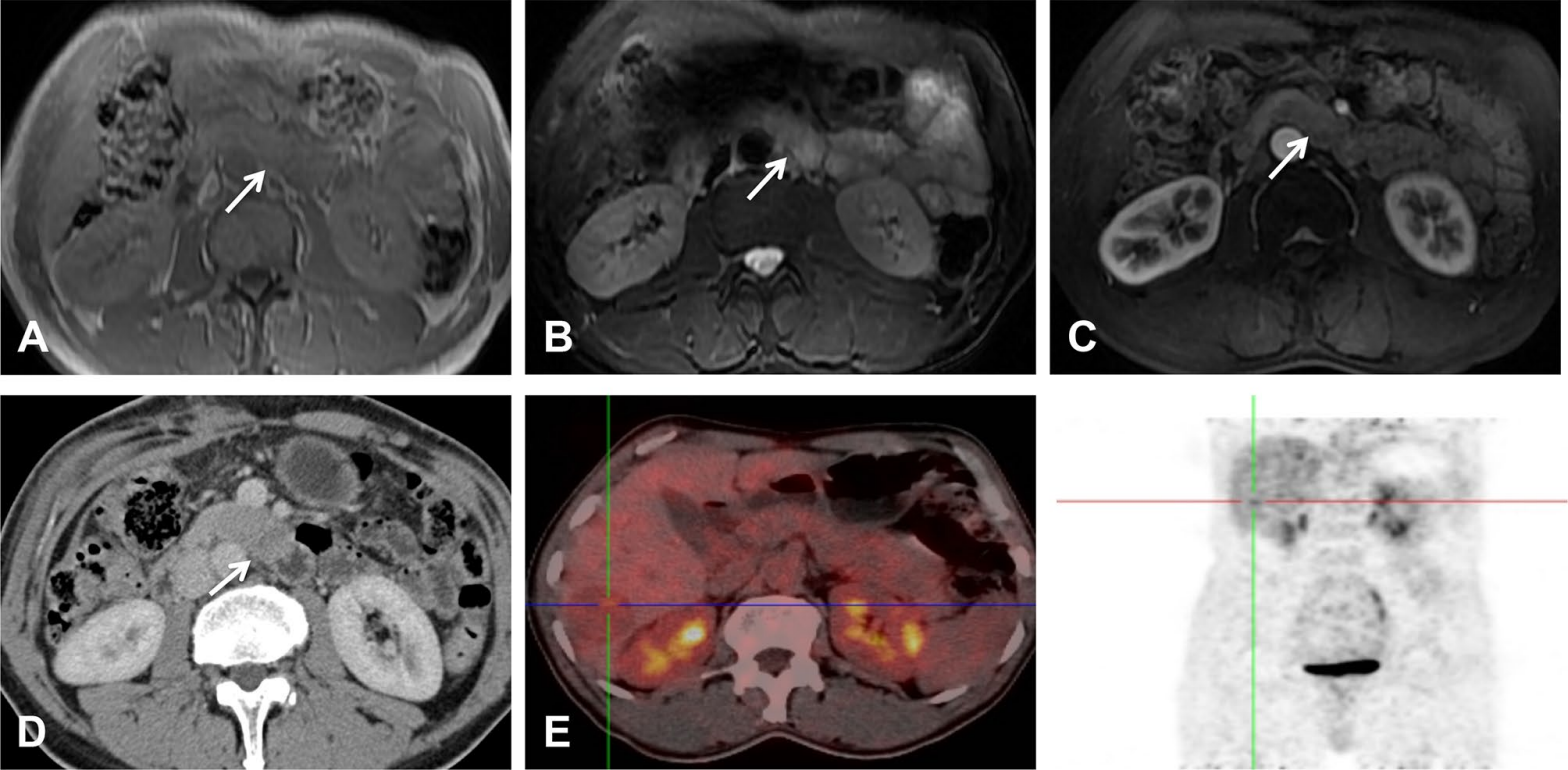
MRI, CT and ^18^F-FDG PET/CT images of a 37-year-old man with PB and multiple liver metastases. The exogenous mass in the uncinate process of the pancreas was isointense on T1WI (A) and hyperintense on axial fat-suppressed T2WI (B). In the AP, the enhancement of the tumors was lower than that of the surrounding normal pancreas (C). The above imaging findings of the tumors are similar to those of the surrounding intestines. On the PET/CT image (E), there was no radioactive uptake in the pancreatic tumor, and only one of the multiple metastatic lesions in the liver showed focal uptake (cross-hairs). (D) One month after the MRI scan, the CT scan revealed a cylindrical exophytic neoplasm of the uncinate process of the pancreas, similar to branches growing outward from a tree trunk.
